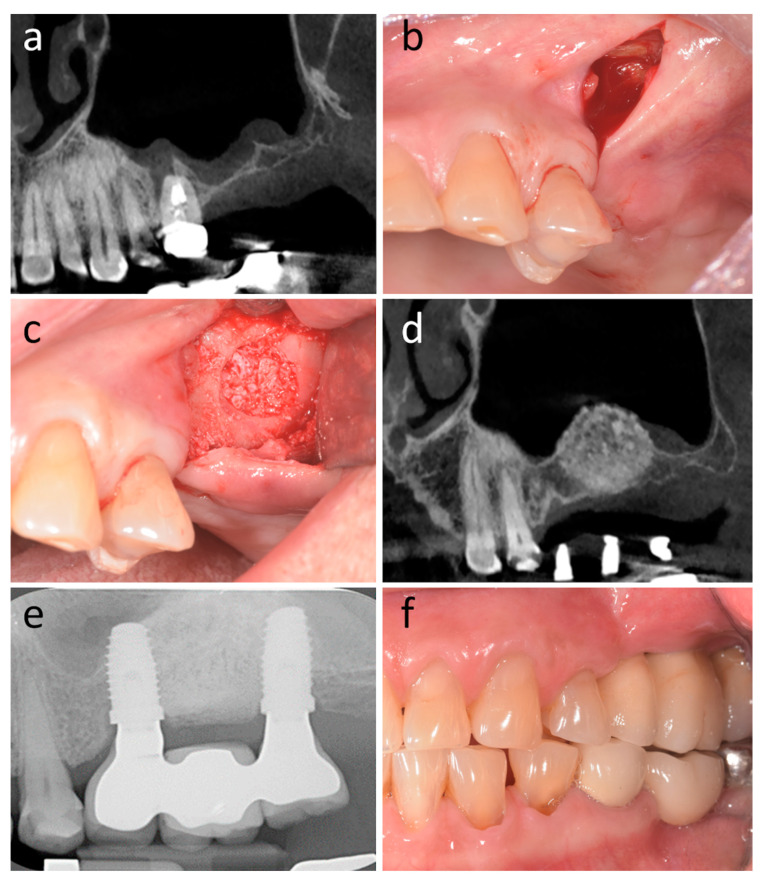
Representative clinical case of tunnel flap application belonging to the author’s (S.S.) original research data. (a) Preoperative CT demonstrating right upper alveolar process atrophy associated with edentulism. (b) Single buccal incision mesial to the antrostomy area. (c) Full-thickness flap elevated and heterologous bone graft in place at the end of the procedure. (d) Postoperative CT scan at 5 months with the graft in place. (e) Implants placed in the bone graft and prosthesis in place. (f) Intraoral view of the prosthesis on implants.
